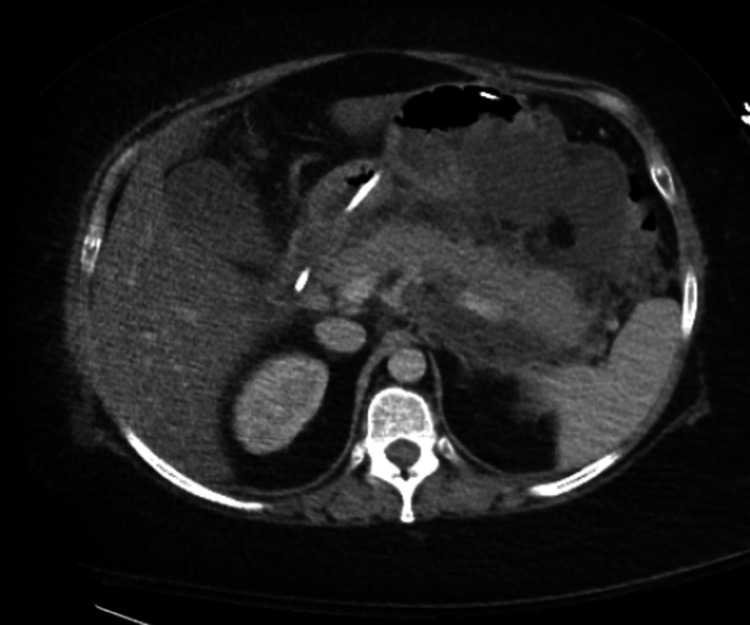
CT evidence of acute pancreatitis with peri-pancreatic necrosis.
